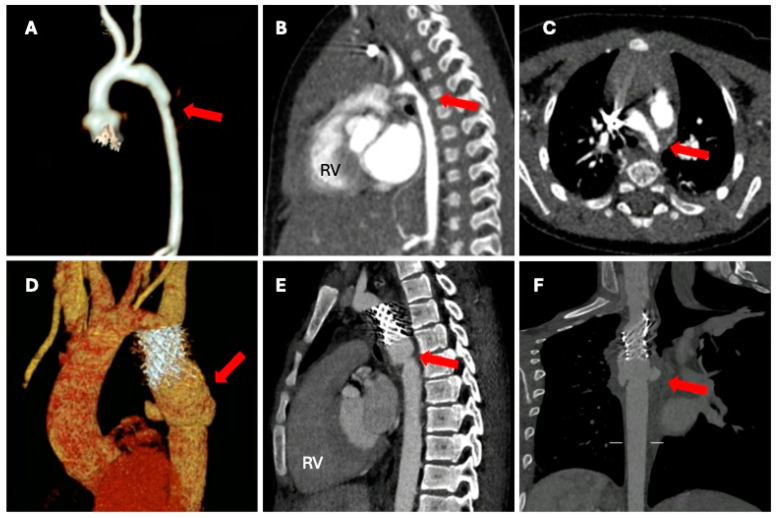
A 4-month-old infant presenting a CoAo at birth. Post-surgery volume rendering post-processed CCT images showing the surgery result (Panel (A)), preoperative sagittal CCT images showing severe CoAo (Panel (B)), and axial preoperative CCT images (Panel (C)). A 17-year-old boy presenting a CoAo and postoperative pseudo aneurysm formation. Volume rendering images (Panel (D)), sagittal visualization at the multiplanar reconstruction (Panel (E)), and linear multiplanar reconstruction (Panel (F)). Legend: Right ventricle (RV); cardiac computed tomography (CCT); coarctation of the aorta (CoAo).
